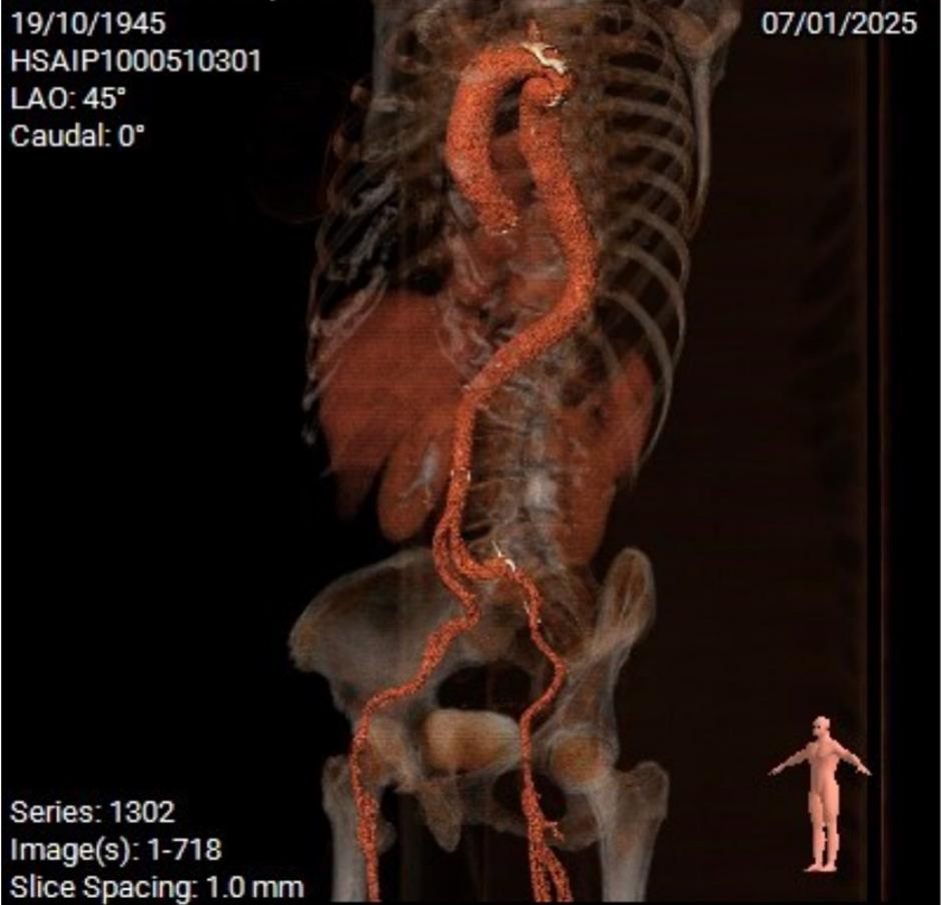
CT scan reconstruction of the aorta indicating pseudocoarctation.
